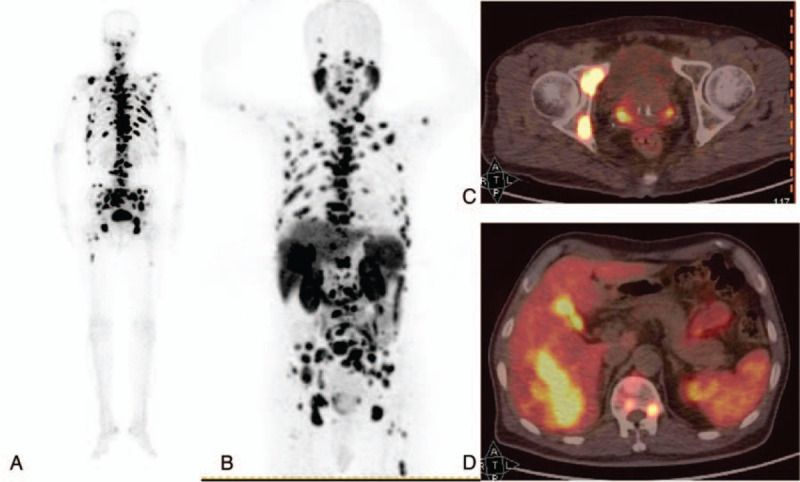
^99m^Tc-MDP whole-body bone scanning and ^18^F-PSMA-1007 PET/CT imaging of the patient (gender: male, age: 77 years) with PC accompanied by primary HCC. (A) ^99m^Tc-MDP imaging suggests multiple bone metastases in the whole body; (B) ^18^F-PSMA-1007 PET MIP imaging suggesting multiple hypermetabolic lesions in the liver, bone, prostate, and lymph nodes; (C) ^18^F-PSMA-1007 PET/CT fusion imaging suggesting localized high metabolite levels within the bilateral peripheral lesions of the prostate with an SUVmax of 11.7; D,^18^F-PSMA-1007 PET/CT fusion imaging suggesting a high metabolite lesion in the right lobe of the liver with an SUVmax of 27.5. ^18^F = Fluorine, HCC: hepatocellular carcinoma, PC: prostate cancer, PET/CT: positron emission tomography/computed tomography, PSMA: prostate-specific membrane antigens, SUVmax: maximum standardized uptake value.
